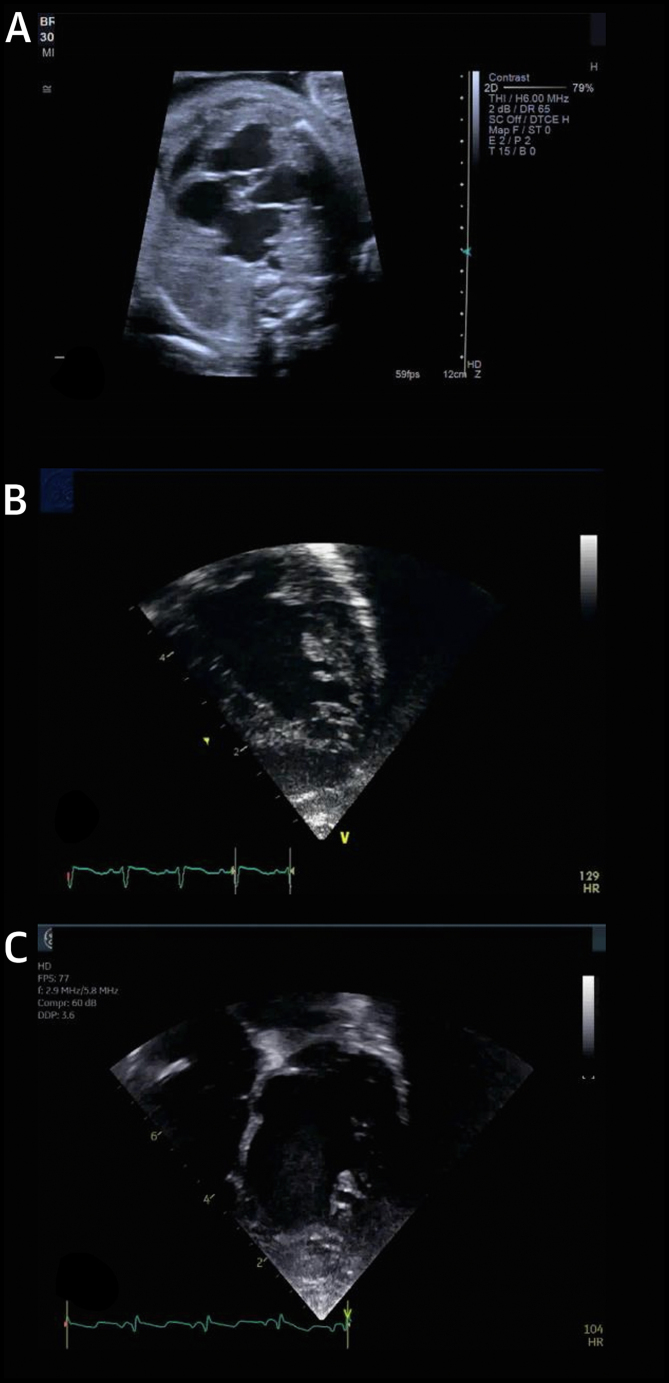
Case Report 2 (A) Fetal echocardiogram at 25 weeks in a child with hydrops fetalis demonstrated severely depressed biventricular systolic dysfunction with excessive trabeculation (Video 3). (B) Supraventricular tachycardia in this fetus developed 1 week later. Forty-eight hours after delivery, left ventricular EF reduced to 33% (Video 4). (C) Five years later, the same patient demonstrated progressive left ventricular dilatation with an left ventricular end-diastolic diameter *Z* score of +2.7 and borderline EF of 50% and global longitudinal strain of −17% (Video 5). The working diagnosis was cardiomyopathy with excessive trabeculation. Neonates and children with excessive trabeculation have been understudied, with low rates of longitudinal follow-up. Such patients should routinely undergo follow-up, with close clinical evaluation and potentially neuromuscular disease testing. If familial disease is suspected, genetic testing may be indicated. Abbreviation as in Figure 4.
